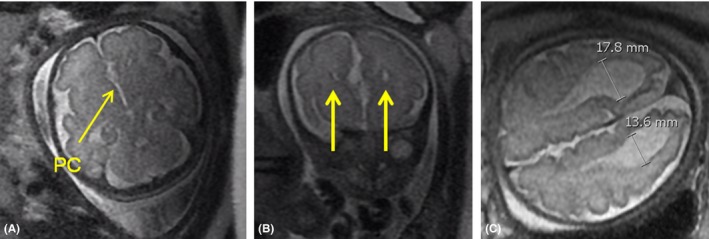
Fetal MRI at 31w3d gestation (single shot fast spin‐echo T2‐weighted images). The corpus callosum is not visualized on any images. A, Axial image demonstrates an abnormal interhemispheric fluid collection anteriorly which does not have morphology consistent with cavum septi pellucidi (CSP) and is therefore a pseudocavum (PC). B, Coronal image demonstrates trident‐shaped frontal horns of the lateral ventricles (arrows). C, Axial image shows dilation of the occipital horns of the lateral ventricles with teardrop configuration consistent with colpocephaly
